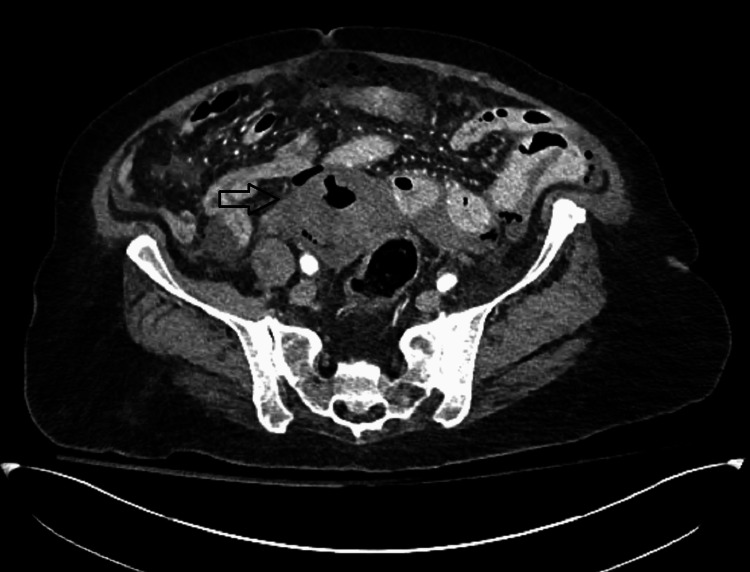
Axial cut from CT abdomen/pelvis showing intraperitoneal and periuterine free air
